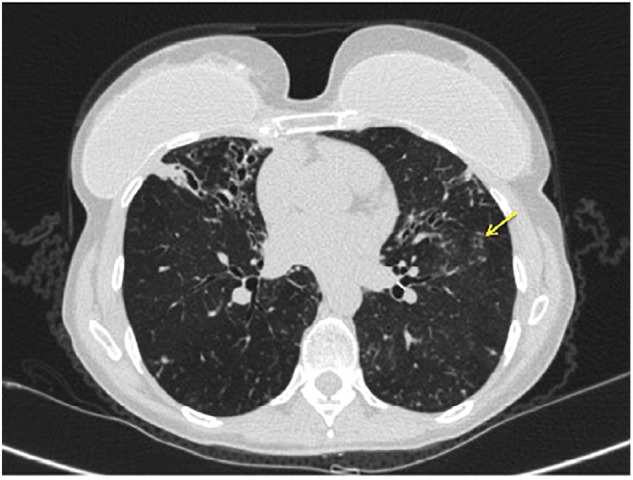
Axial CT chest image demonstrating bronchiectasis and mucoid impaction in the right middle lobe. The tree-in-bud nodularity in the left lung (yellow arrow).
